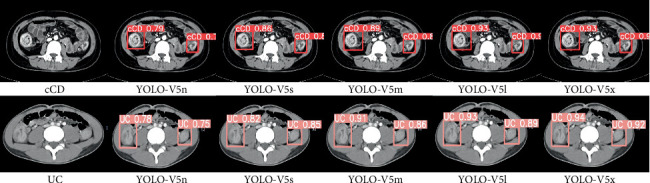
The results of five submodels of YOLOv5 in the detection and classification of cCD (top row) and UC (bottom row) on computed tomography enterography (CTE) images. The CT signs of those two cases were very similar on CTE images. YOLOv5n obtained the lowest confidence score among the submodels, while the confidence score of YOLOv5x was the highest.
